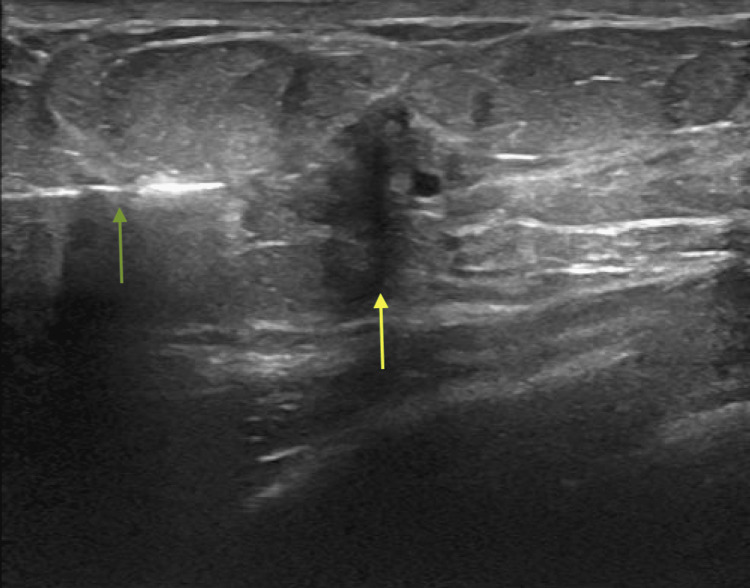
Ultrasound-guided biopsy of the six o'clock mass Green arrow: biopsy needle Yellow arrow: mass
